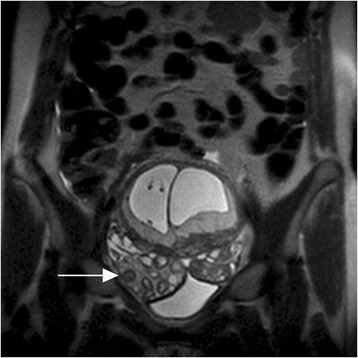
Ovarian torsion with stimulation. Coronal T2-weighted image in a 31-year-old woman with right lower quadrant pain at 11 weeks gestational age (dichorionic diamniotic twin gestation) demonstrates bilateral ovarian enlargement with asymmetric enlargement of the right ovary (arrow) and increased T2 signal intensity of the right ovarian stroma. Right ovarian/tubal torsion was surgically detorsed
